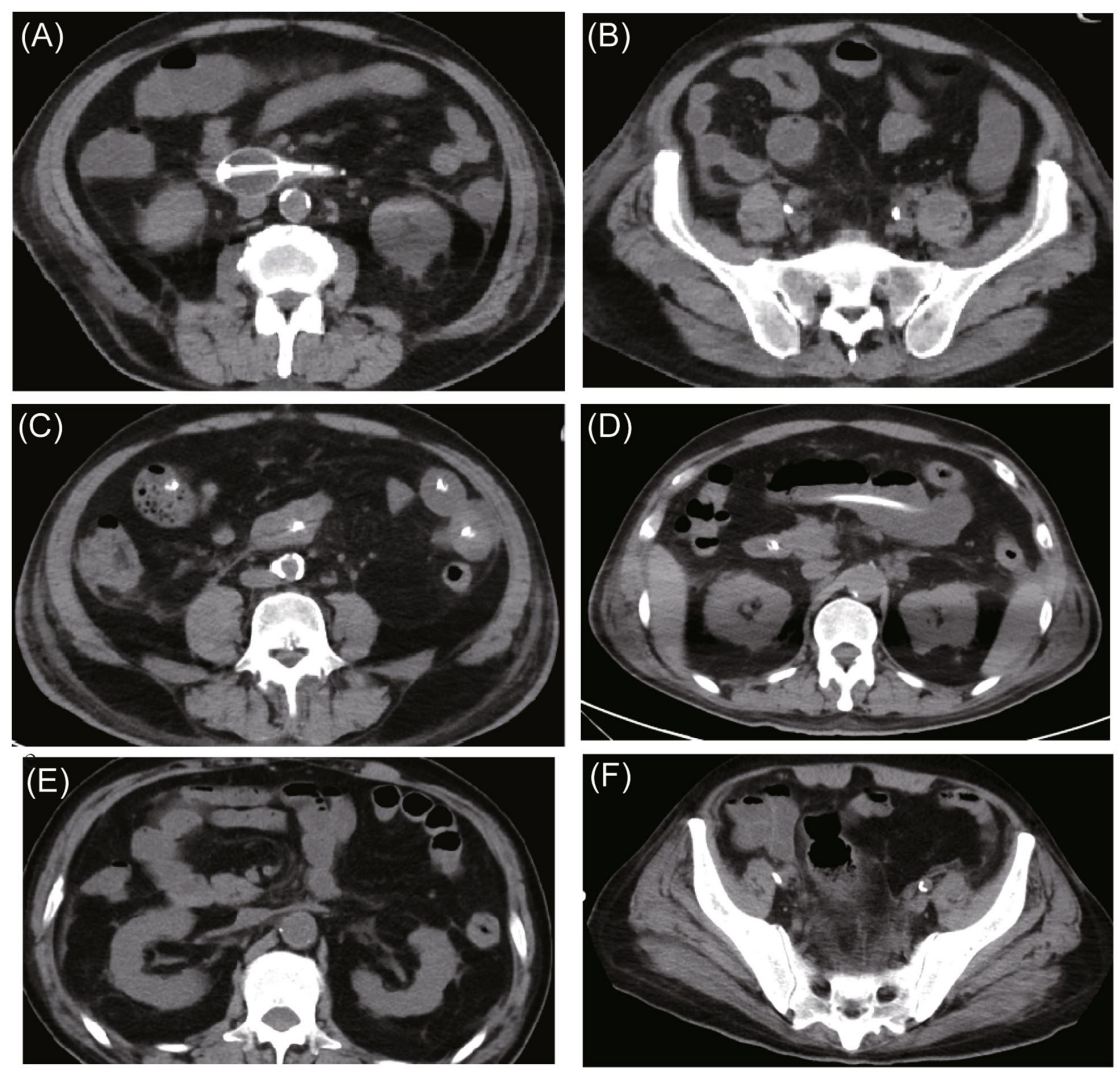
Serial abdomen and pelvic CT scans demonstrating the evolution of intestinal edema and fluid accumulation. (A,B) On hospital day 3, thickening of the perirenal fascia are observed. The colon and segments of the small intestine show wall thickening and edema with intraluminal fluid accumulation. A nasogastric decompression tube is in place, draining large volumes of gas and yellowish fluid. (C,D) On hospital day 11, post-decompression changes are evident, with partial resolution of small-bowel wall edema. Residual gaseous and fluid distension of the small intestine is present, along with colonic and rectal dilatation containing both gas and fecal material. (E,F) On hospital day 25, marked improvement in small-bowel gaseous and fluid distension is seen. However, increased fat stranding around the sigmoid colon persists, suggestive of residual inflammatory changes.
